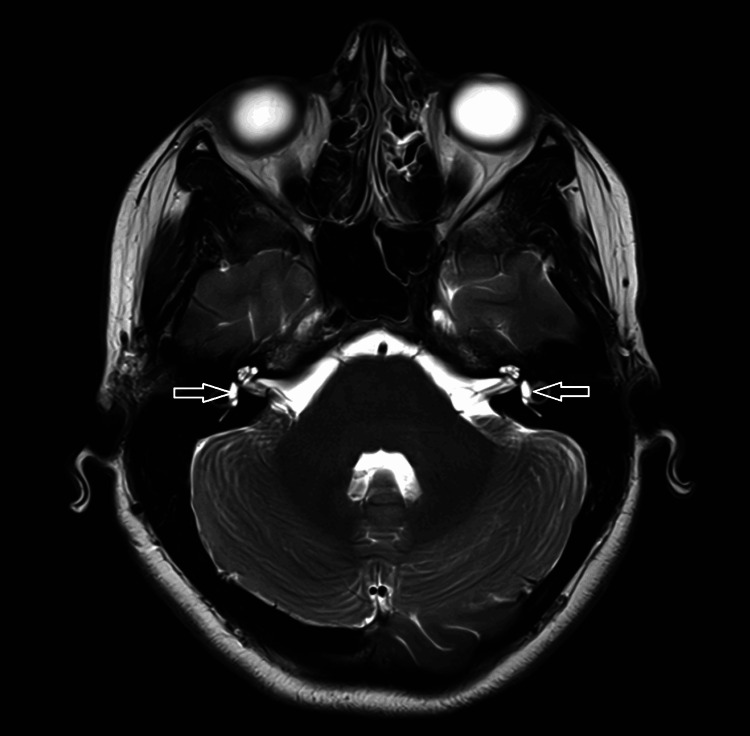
MRI brain showing bilateral facial nerve enhancement (arrows)
